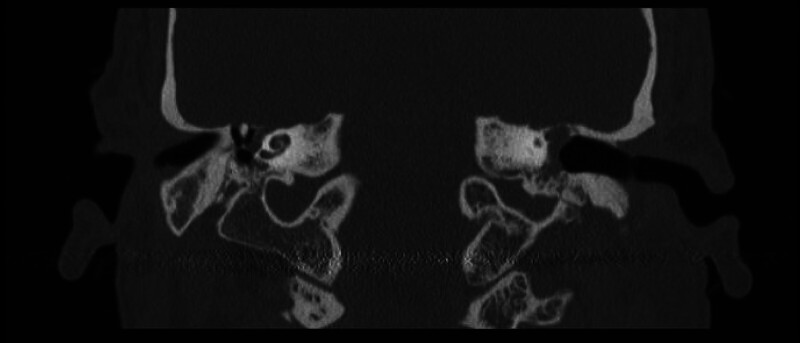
Preoperative computed tomography showing tegmen defect on the right side with the malleus above the floor of the middle fossa.
